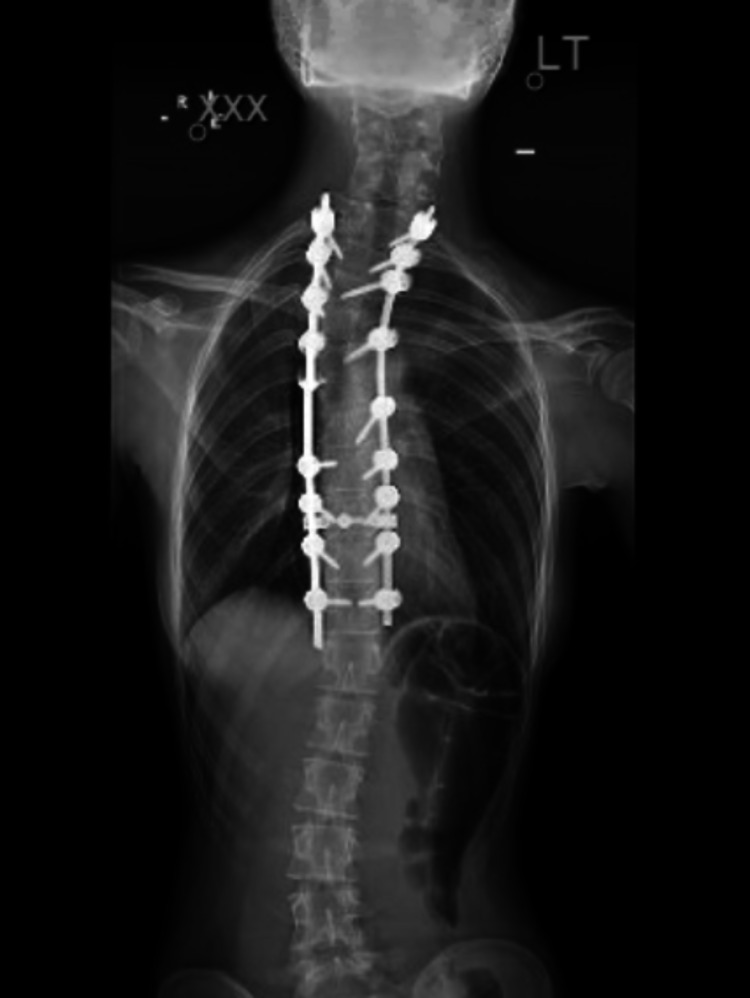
Plain AP radiograph of the patient showing posterior instrumentation for correction of scoliosis and correction of Sprengel's deformity* *The spines of both scapulae can be noticed at the same level AP: anteroposterior
